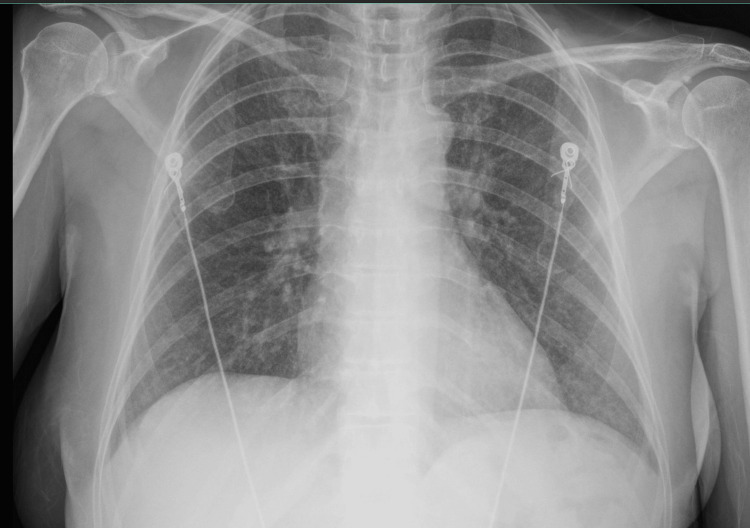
Chest X-ray showing bilateral pulmonary interstitial infiltrate
